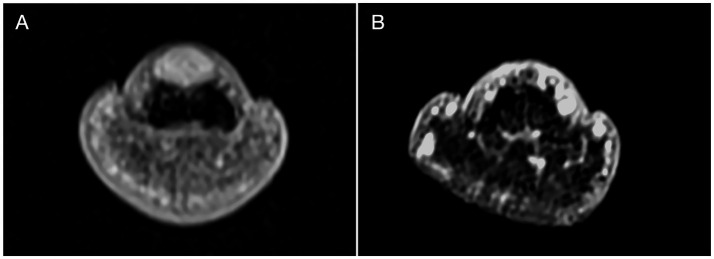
MRI imaging. (A) The T1W1 showed a well defined high signal dot sized 5x2x2mm was displayed in the subungual area. A depression was noticed on the dorsal part of distal phalanx corresponding to the site of high signal. Signal was more significant than T1W1 and relatively evenly distributed after enhancement. (B) MR investigation on 24 months follow up visit displayed no recurrence and noticeable recovery of the distal phalanx.
